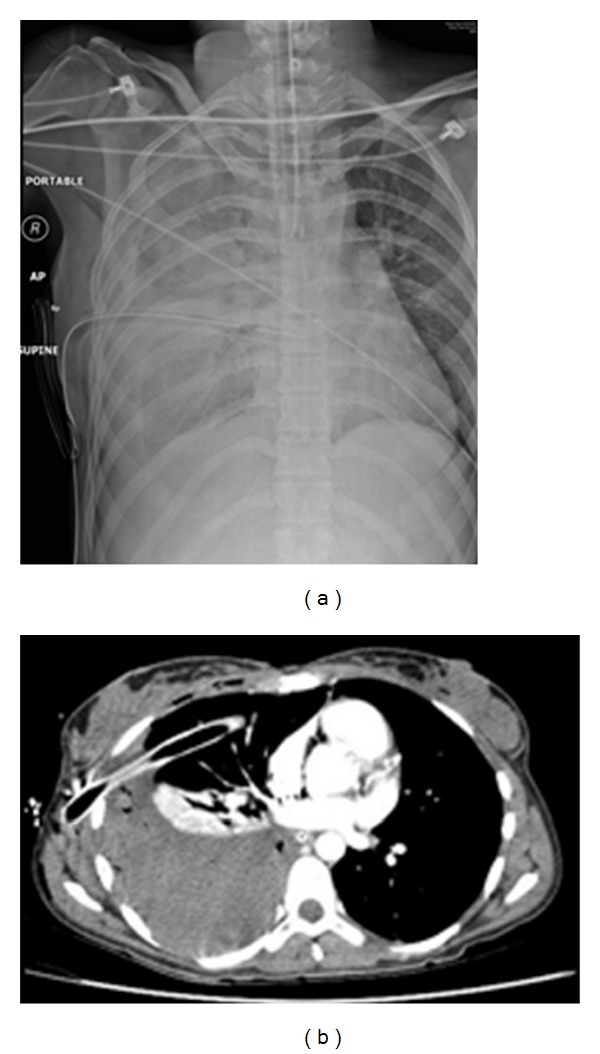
A CXR and axial CT show an inappropriately placed chest drain in an attempt to drain a right basal pleural effusion.
